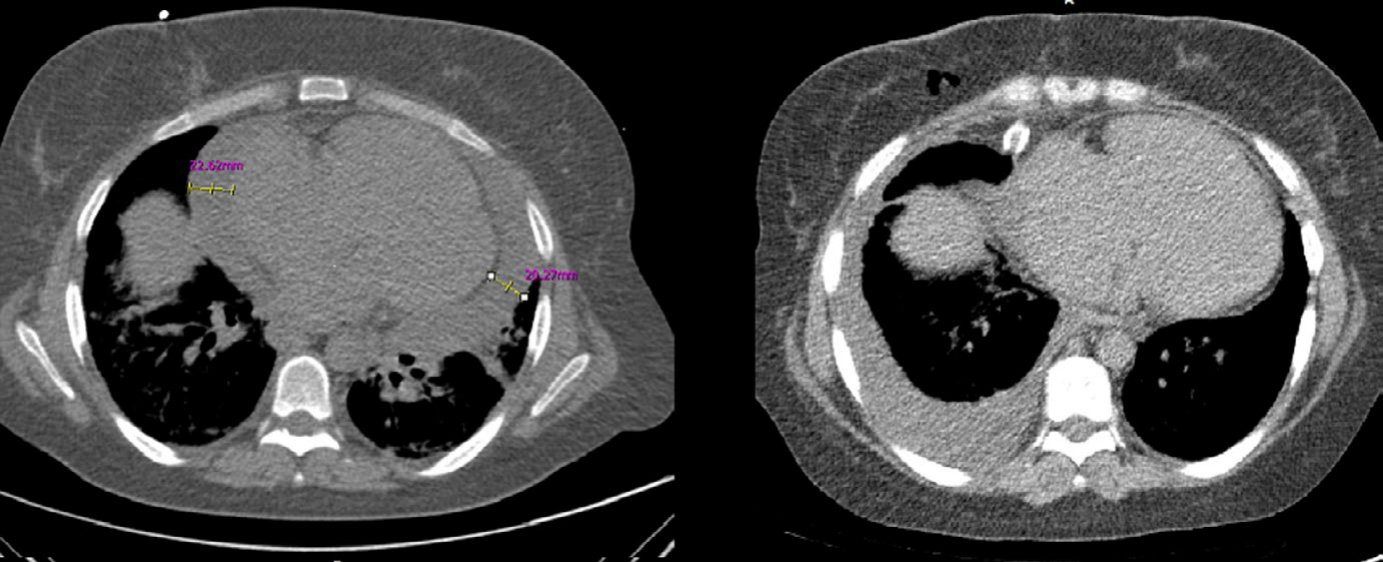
Spiral chest CT scan. Left side: before surgical pericardiocentesis: massive circumferential pericardiocentesis maximum size posterior to LV 25 mm and around RA 24 mm. Right side: after pericardiocentesis: minimal residual pericardial effusion. Abbreviations: CT, Computed tomography; LV, left ventricle; RA, right atrium
